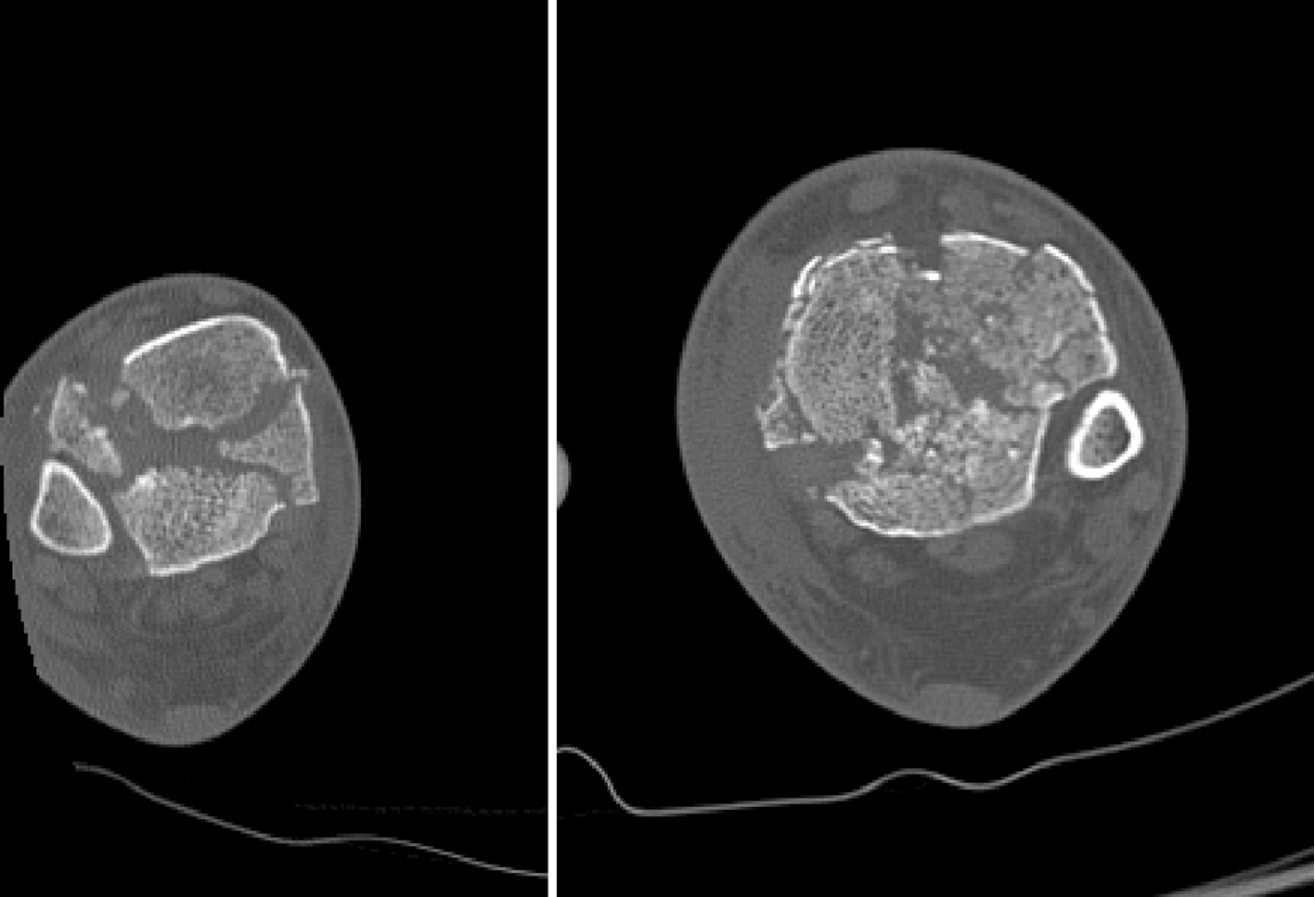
Articular fragments with varying degrees of comminution as seen on axial CT views
